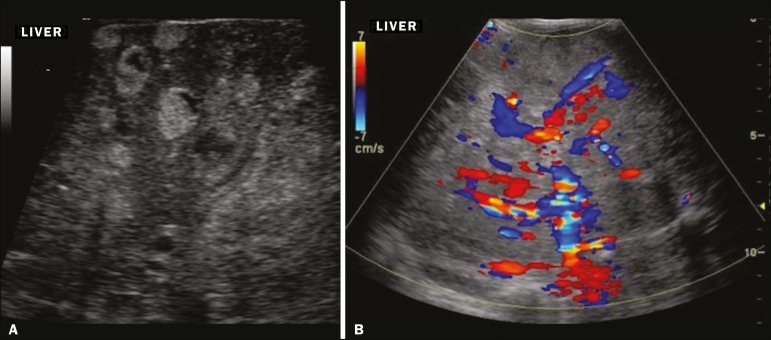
Childhood hepatic hemangioma-diffuse lesions in a 6-month-old girl. A: B-mode ultrasound shows multiple diffusely distributed hyperechoic liver nodules, some with small cystic areas. B: Color Doppler study showing a diffuse increase in hepatic vascularization.
